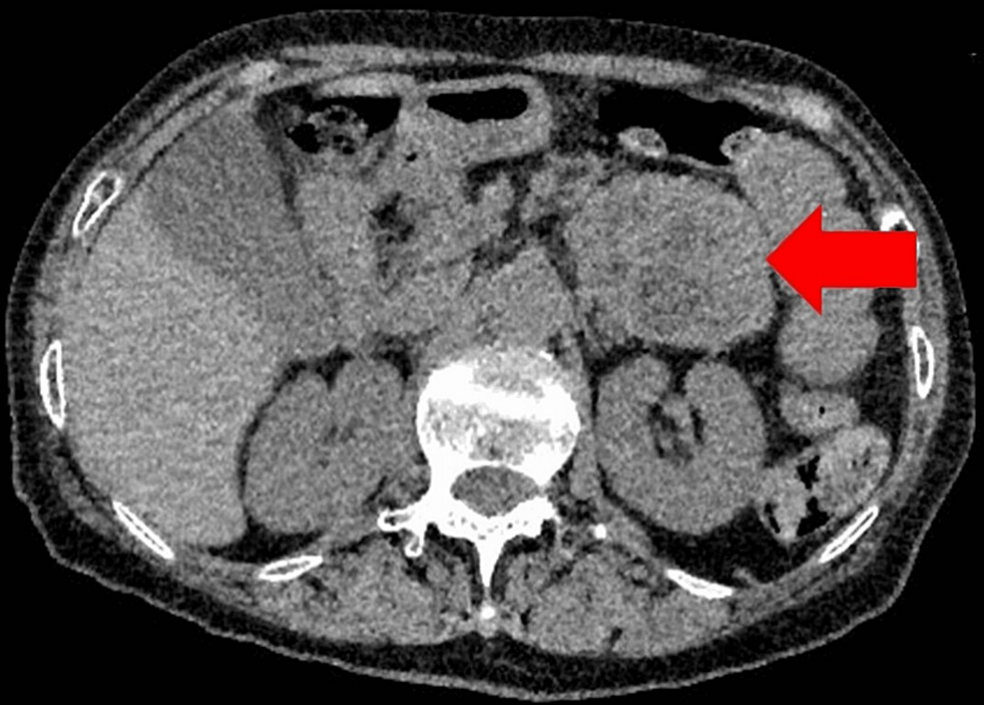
Computed tomography scan 60 x 40 mm solid mass with calcification arising from the pancreatic tail.
